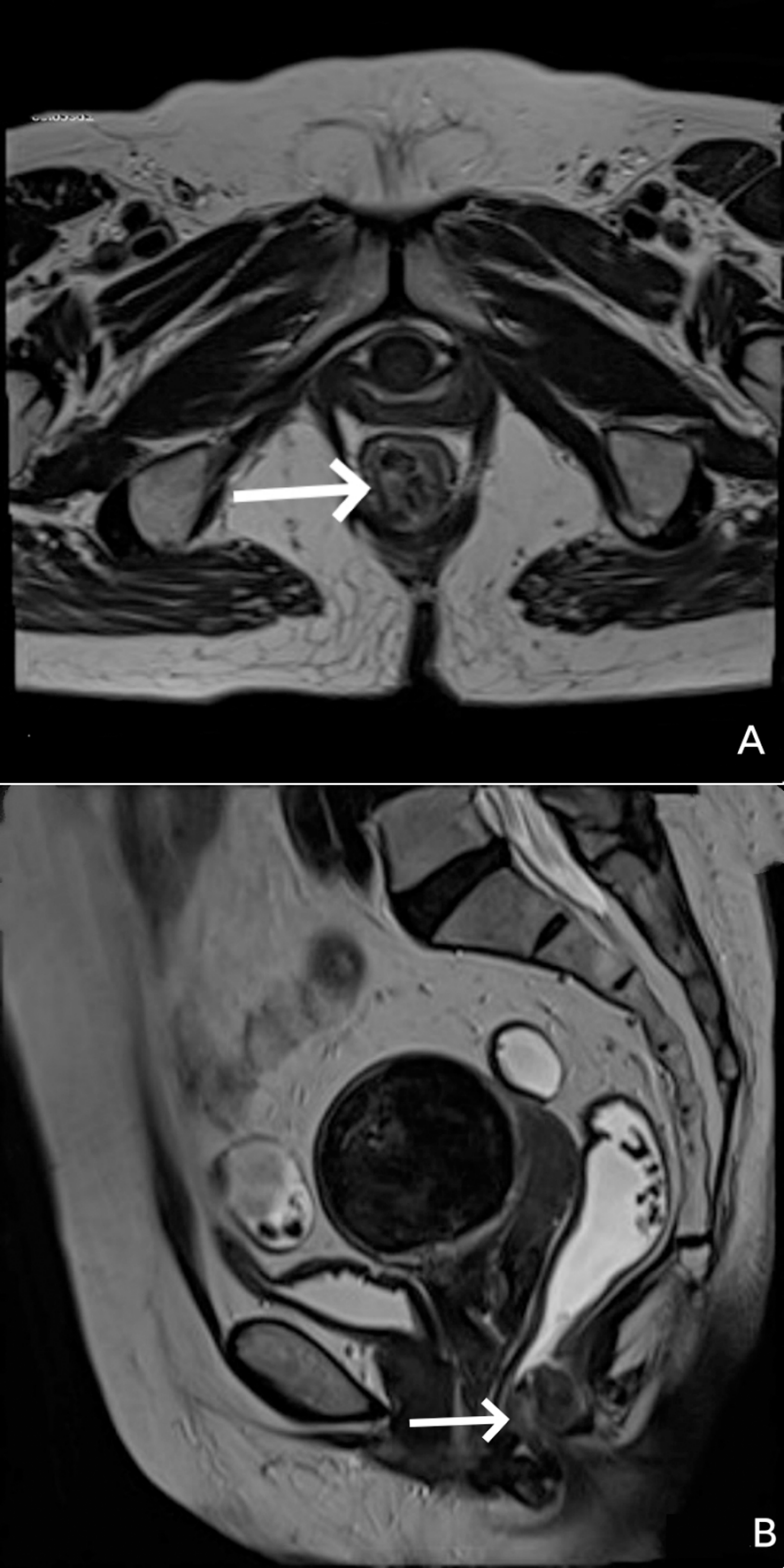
(A) Axial and (B) Sagittal T2-weighted MRI images demonstrating a T2-hyperintense tumor confined to the rectal wall.
